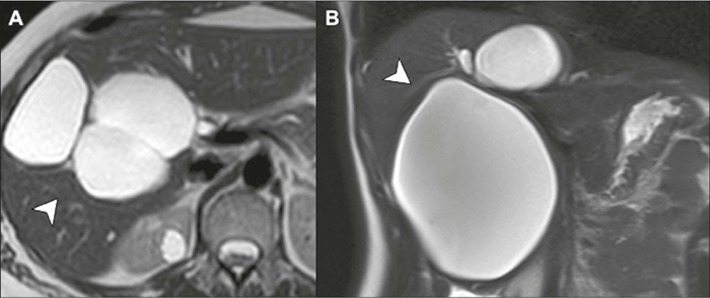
Simple epithelial cysts. T2-weighted MRI in the axial and coronal
